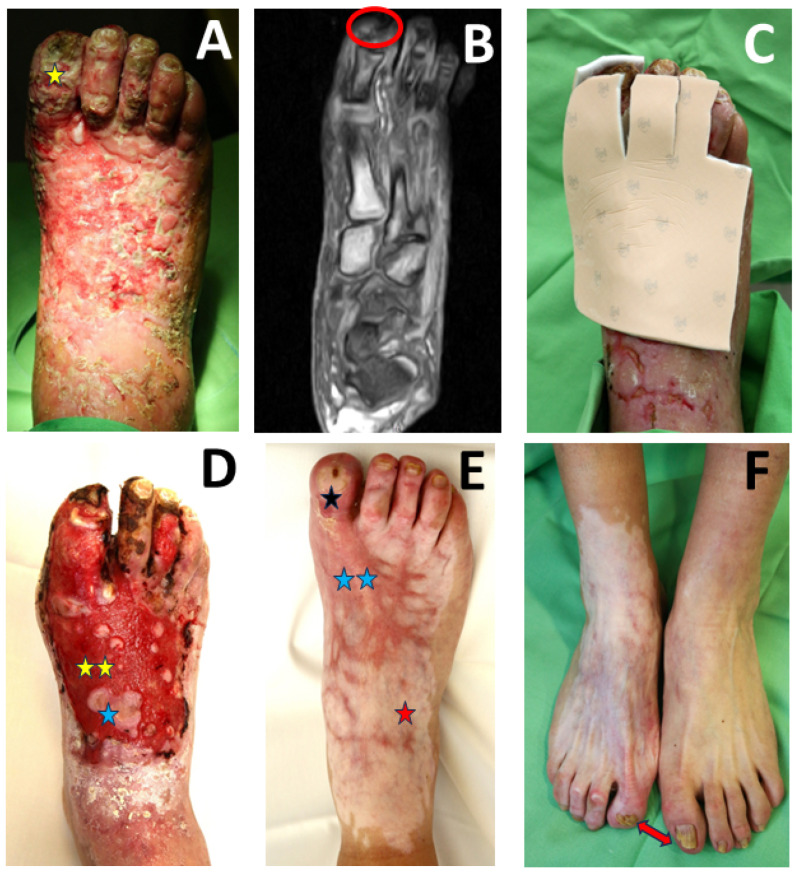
(A) Status at admission included necrosis in the nail ablation area on the first toe (yellow star symbol), on the dorsal surface of the foot, and on the II–IV toes of the right leg. (B) Magnetic resonance imaging of the right foot showed typical symptoms of osteomyelitis, bone marrow edema, and blurred bone contours in the distal part of the distal phalanx of the first toe (red circle). (C) After necrectomy, wound healing was enhanced with conservative treatment with a silver-containing bandage. (D) Granulation (two yellow stars) and epithelization (blue star symbol) stage of wound healing. (E) The ulcer and the deep tissue infection healed in 6 months. (F) The patient was asymptomatic for 18 months after the wound healed. The right first toe was shorter because the distal part of the distal phalanx was resorbed due to osteomyelitis. The inflammation resolved, and only a scar (two blue stars), hypopigmentation (red star symbol), and nail aberrations (black star symbol) remained. The red arrow indicates the shortened first right toe due to bone resorption.
